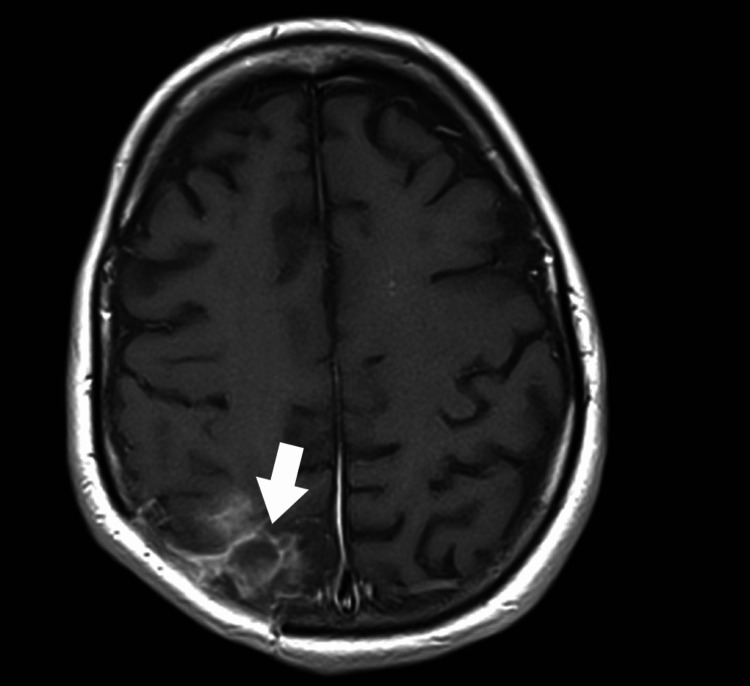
A follow-up MRI scan of the head revealed a residual postoperative change located in the right parieto-occipital area: a top view (marked with a white arrow).
